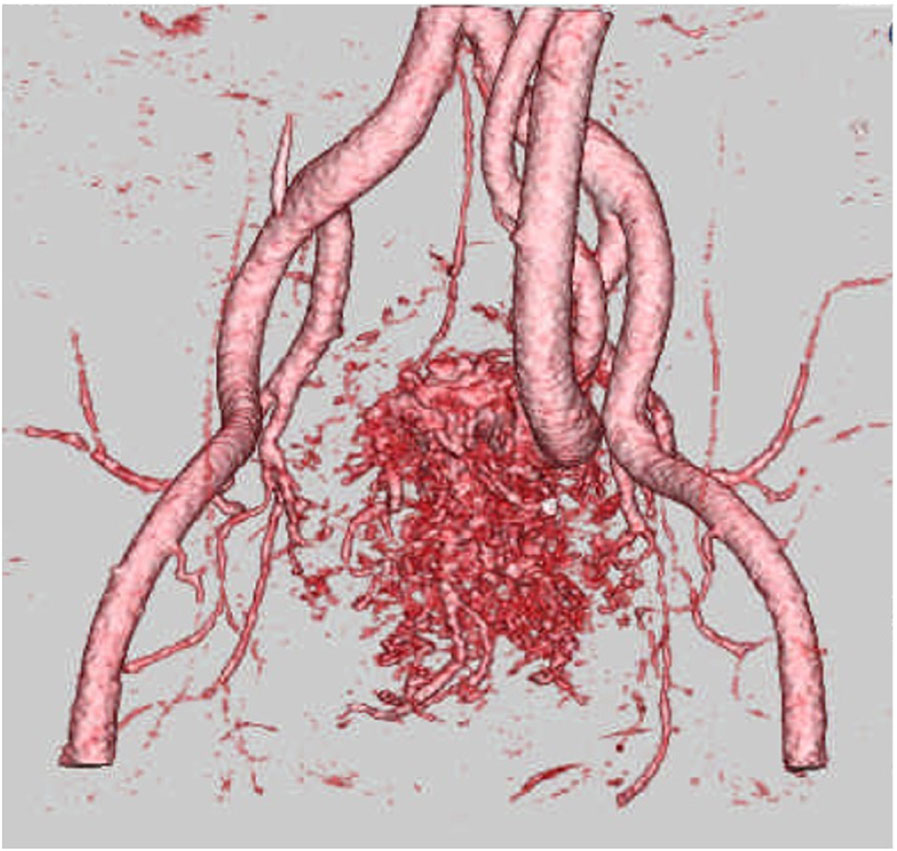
Four-dimensional CT angiography demonstrated that tortuous and dilated IMV supplied blood flow through many fistulas from superior mesenteric artery, bilateral internal iliac artery, and median sacral artery
